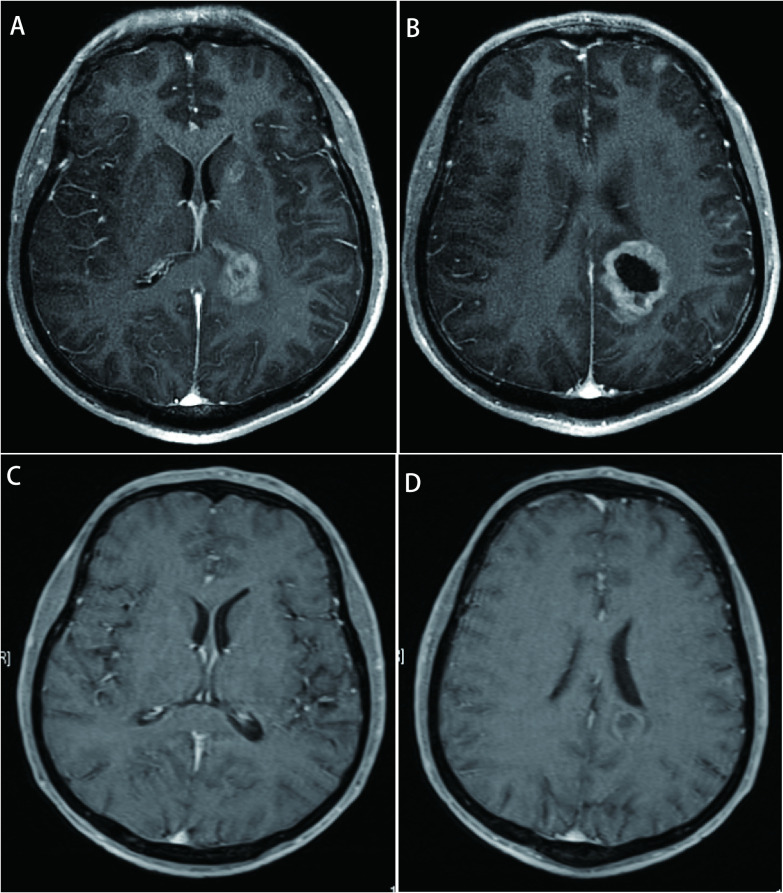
头MRI所示转移瘤情况。A：头MRI(2019年8月6日)：左侧基底节区，左侧脑室体旁转移瘤; B：头MRI(2019年8月6日)：左侧额叶皮层，左侧顶叶皮层，左侧脑室体旁转移瘤; C：头MRI(2020年1月20日)：左侧基底节区缩小，左侧脑室体旁转移瘤缩小; D：头MRI(2020年1月20日)：左侧额叶皮层，左侧顶叶皮层，左侧脑室体旁转移瘤缩小。 Brain MRI showing metastatic tumors. A: brain MRI (6-Aug-2019): metastatic tumor in left basal ganglia region, metastatic tumor near the body of the left lateral ventricle; B: brain MRI (6-Aug-2019): metastatic tumor in left frontal cortex, metastatic tumor in left parietal cortex, metastatic tumor near the body of the left lateral ventricle; C: brain MRI (20-Jan-2020): metastatic tumor in left basal ganglia region was reduced, metastatic tumor near the body of the left lateral ventricle was reduced; D: brain MRI (20-Jan-2020): metastatic tumor in left frontal cortex was reduced, metastatic tumor in left parietal cortex was reduced, metastatic tumor near the body of the left lateral ventricle was reduced. MRI: magnetic resonance imaging.
